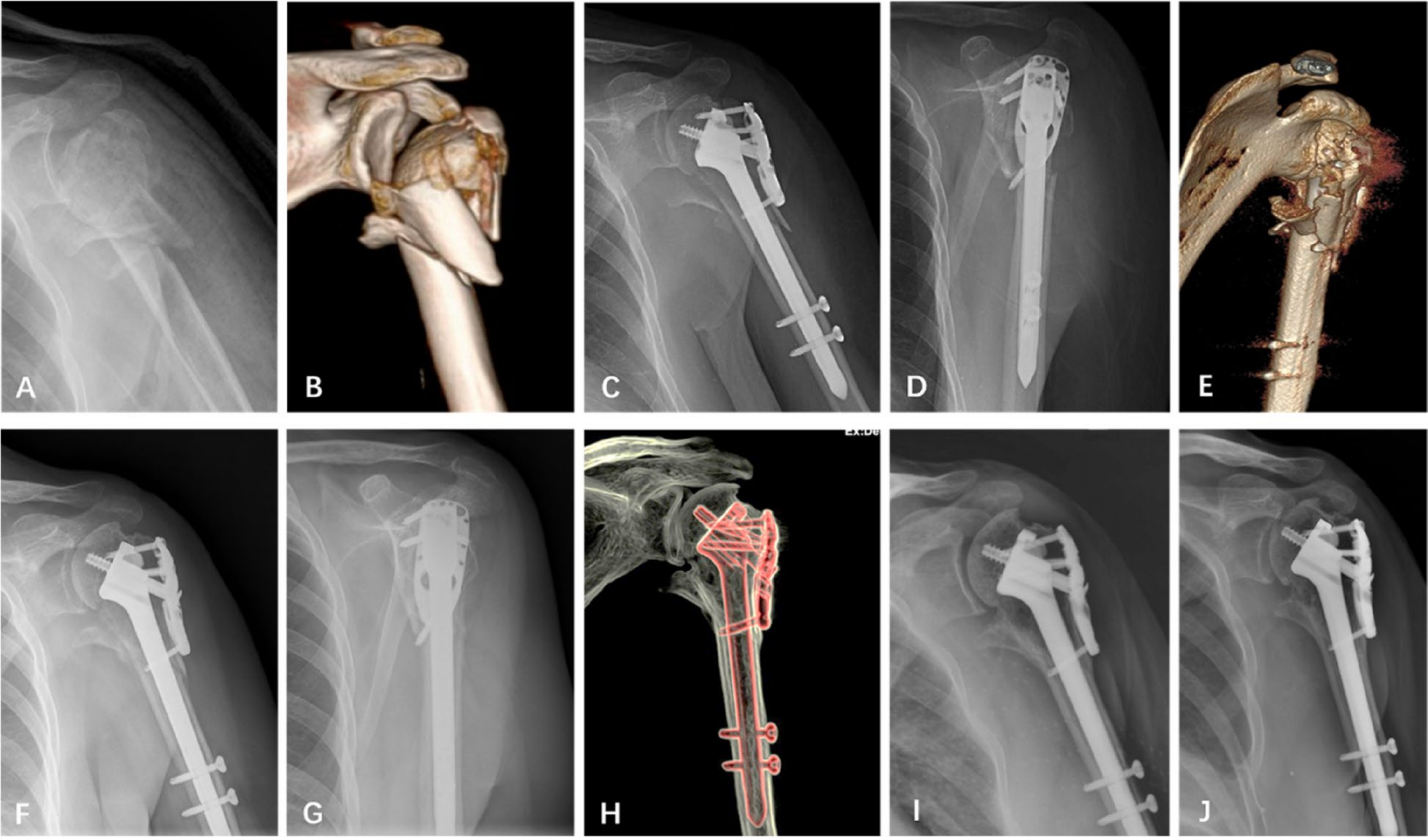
Preoperative anterior–posterior (AP) radiograph (A) and three-dimensional computed tomography (3D CT) reconstruction (B) of a shoulder revealing a 4-part PHF with comminuted medial cortex. Postoperative AP (C) and outlet view (D) radiographs and 3D CT reconstruction (E) of the shoulder revealing favorable head-shaft alignment, despite the medial cortex remaining obviously displaced. Three months postoperatively, the AP (F) and outlet view (G) radiographs and 3D CT reconstruction (H) of the shoulder revealed fracture healing without obvious reduction loss. Six (I) and 12 (J) months postoperatively, AP radiographs of the shoulder revealed firm healing of the displaced medial cortex to the humeral shaft and remolding over time
